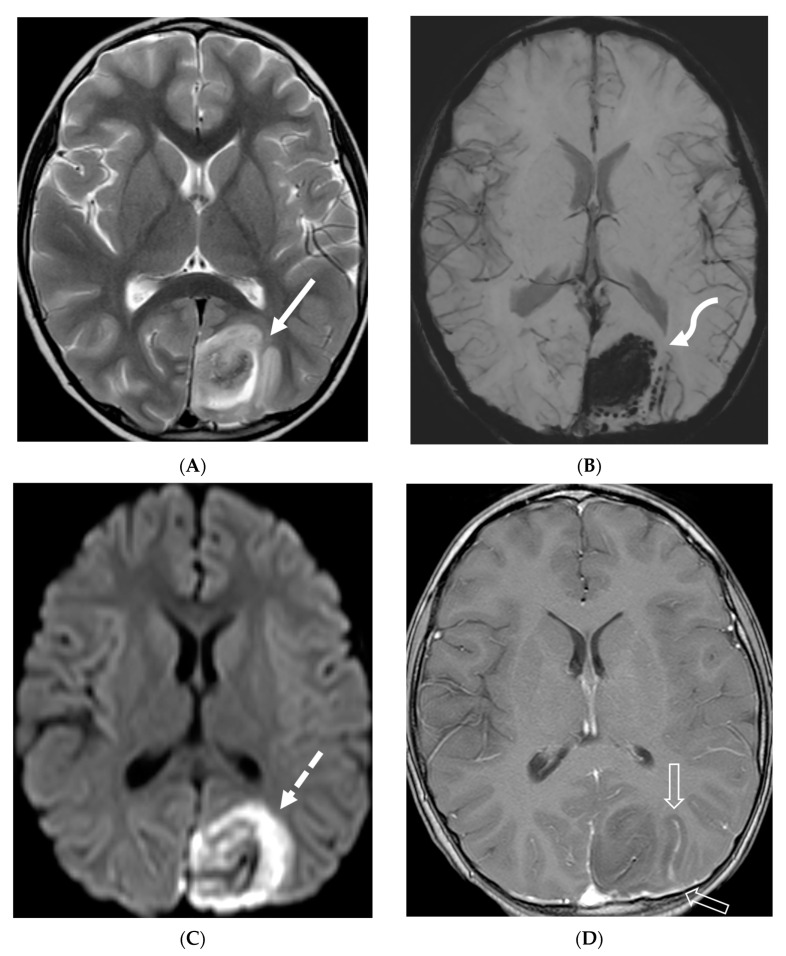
Axial T2 (A), axial DWI (B), axial SWI (C) and axial T1 post contrast (D): 4-year-old girl with acute lymphoblastic leukemia, pancytopenia and fever. Treatment started one week before with asparaginase. There is prominently T2 hyperintensity and swelling of the gyri involving the medial aspect of the left parietal occipital cortex (arrow). Multiple small foci of T2 hypointensities are identified within the involved region with corresponding blooming on the susceptibility indicating hemorrhage (curved arrow) and peripheral rim of true restricted diffusion (dashed arrow). Postcontrast images show pachymeningeal and LME in the involved region (open arrows). Features are concerning for fungal infection. Pathology revealed Rhizomucor pusillis (thermophilic fungus).
